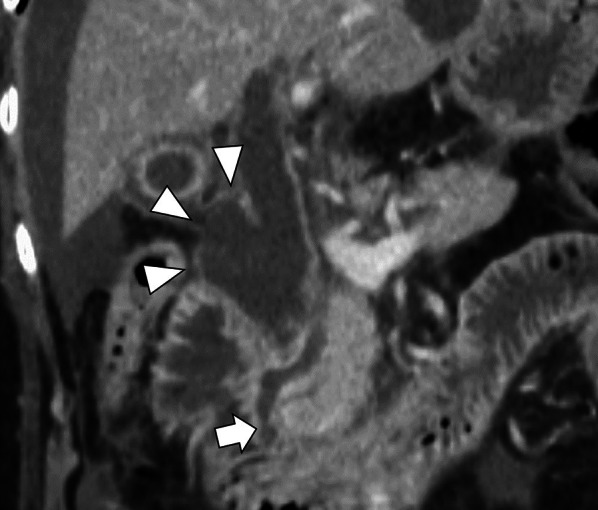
Preoperative computed tomography (coronal view). The common channel of the pancreaticobiliary duct (arrow) was long at 9.3 mm in length and seemed to be joined outside the muscular layer of the duodenal papilla on the enhanced computed tomography coronal view. The dilated common bile duct was accompanied by a diverticulum-like change (arrowhead)
